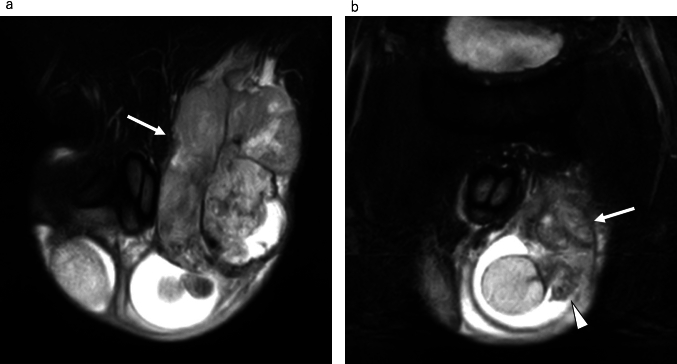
Rhabdomyosarcoma in a 21-year-old male with a left scrotal mass enlargement. a, b Coronal fat-suppressed T2-weighted MR images shows a heterogeneous hyperintense mass (white arrow) located in the spermatic cord. The mass invades the epididymis (white arrowhead), but the testis is preserved
